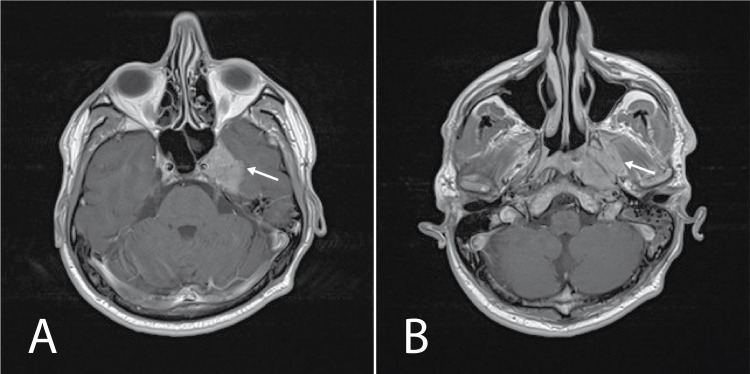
December 2018 initial brain MRI with intravenous contrast. A. a mass with contrast enhancement in the left cavernous sinus, Meckel's cave and medial cranial fossa (arrow) (T1 post contrast sequence); B. a mass with contrast enhancement expanding to pterygoid fossa (arrow) (T1 post contrast sequence).
